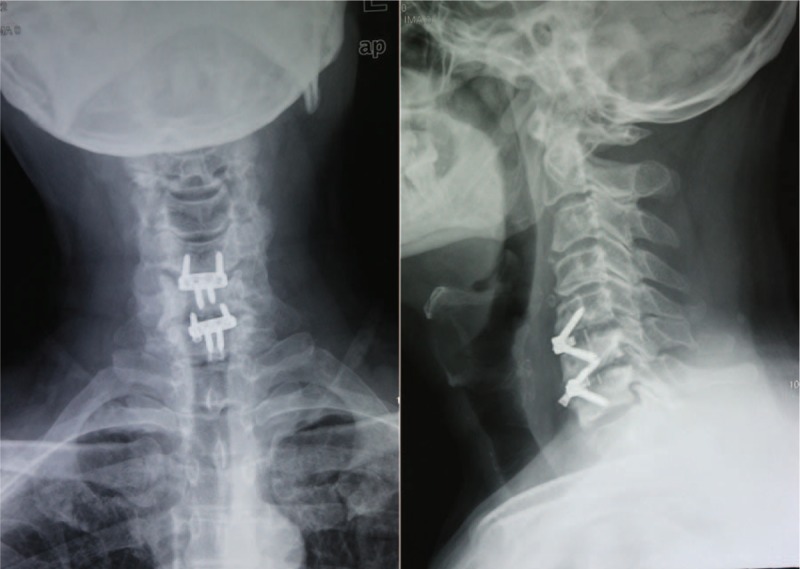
Six months postoperative x-rays showed a locking screw at the segment C6/7 pulled out.
